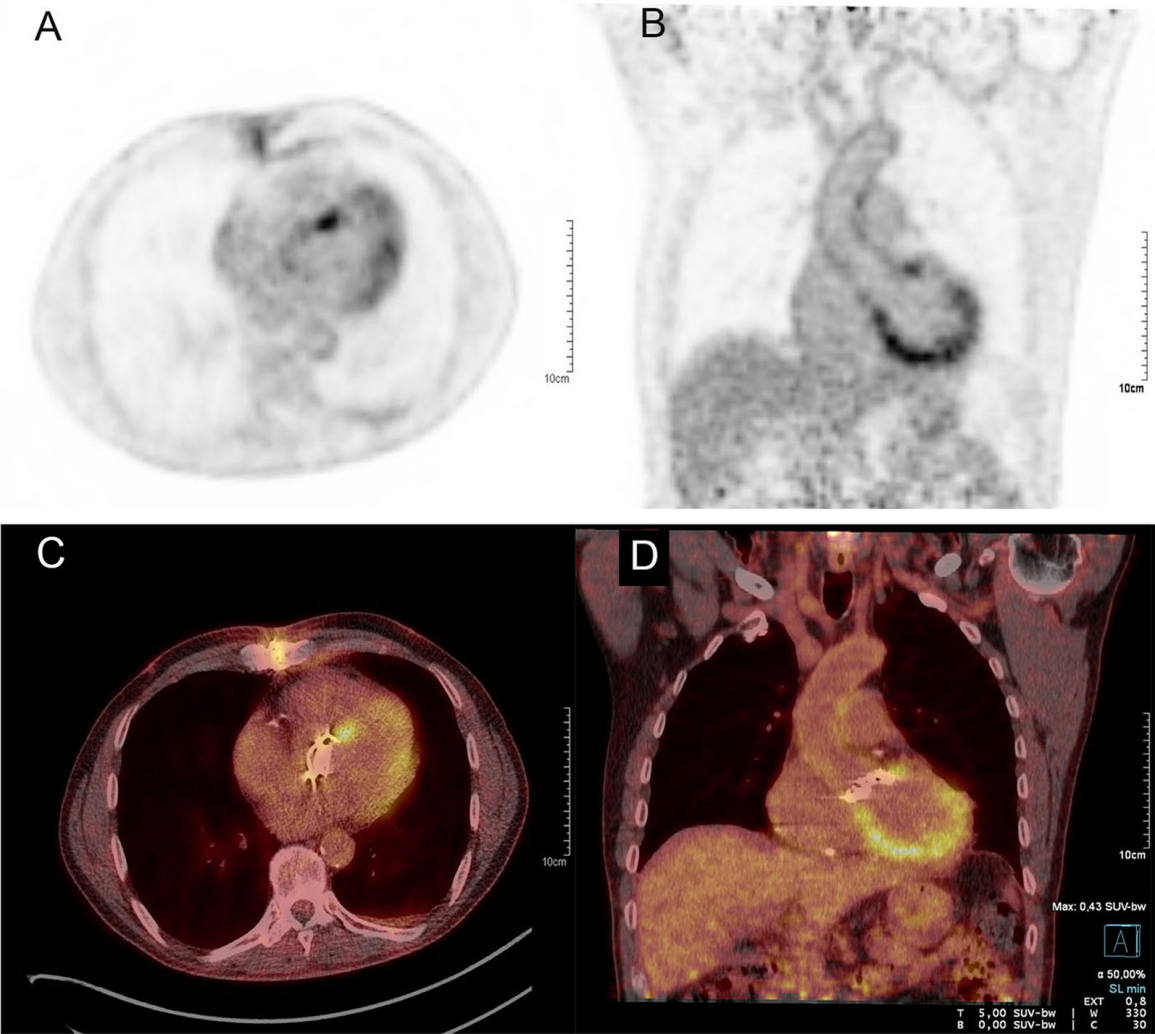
Attenuation-corrected ^18^F-FDG PET images (A, B) and fused images (C, D) of a patient with a high level of myocardial ^18^F-FDG uptake making correct measurements of the SUV values more difficult
